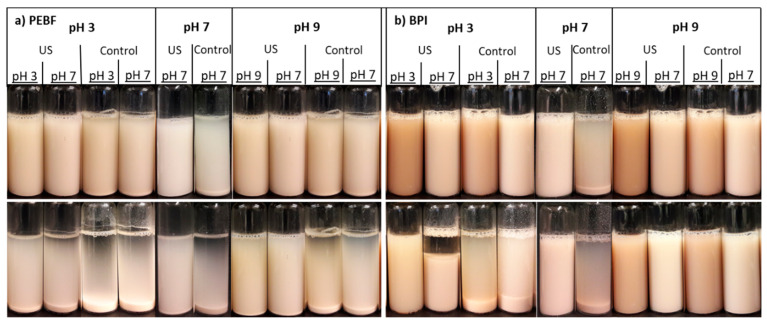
Impact of ultrasound treatment and pH on colloidal stability of (a) protein-enriched barley fraction (PEBF) and (b) barley protein isolate (BPI) dispersions at 1.5% (*w/w*) solid content after 10 min (upper row) and 180 min (lower row) of standing. The first text row indicates pH-value of the ultrasound/control treatment, the second row indicates whether the ultrasound treatment was performed for the sample or not, and the third row shows the colloidal stability analysis pH.
